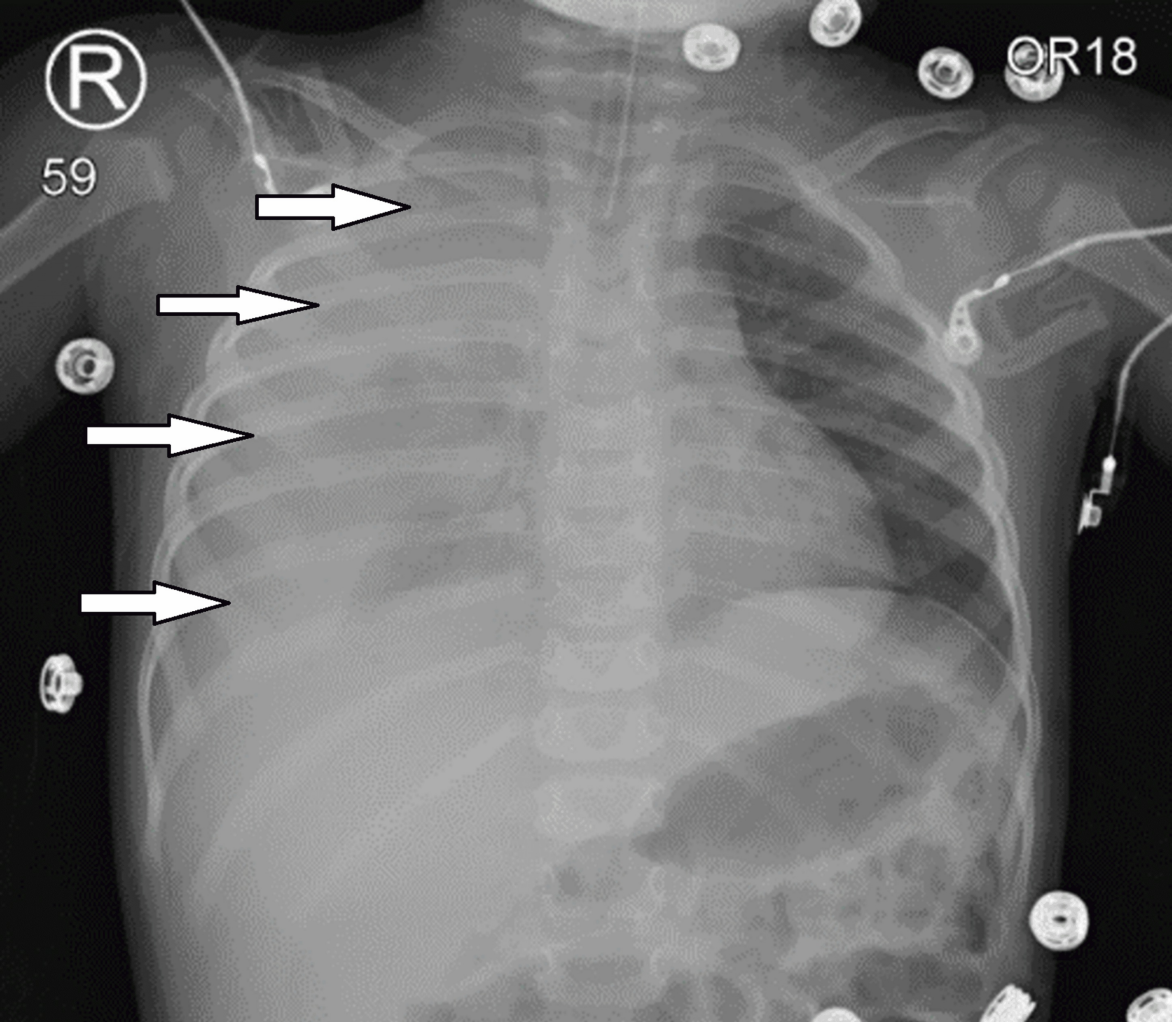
Post-ROSC anteroposterior chest X-ray showing right-sided hemothorax (white arrows). ROSC, return of spontaneous circulation
